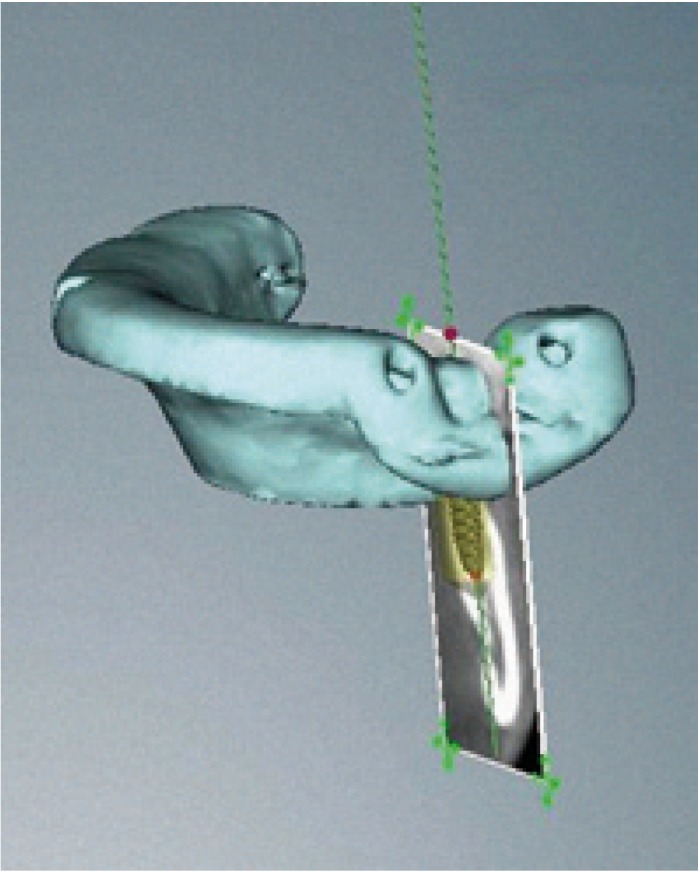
Planning with the NobelGuide® system. Note emergence of the implant in relation to the X-ray splint and the future prosthetic rehabilitation.
